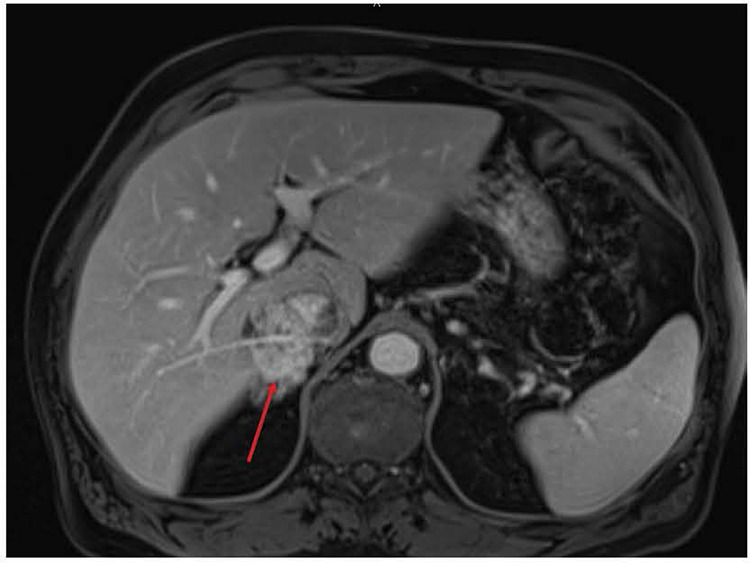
Pre-operative MRI showing involvement of the retro-hepatic IVC by leiomyosarcoma (red arrow). IVC, inferior vena cava.
